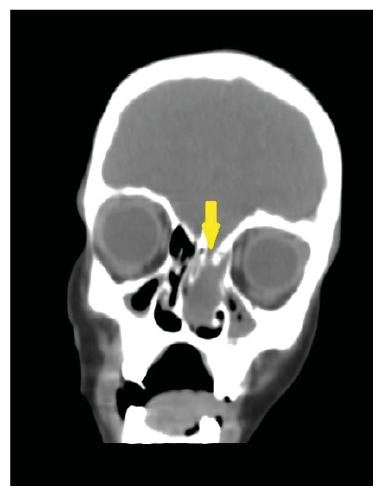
On coronal reformated CT images, a bony defect is seen on left lamina cribrosa. (yellow arrow). Heterogenous image of encephalocele and mucosal tissue is seen on the left ethmoid sinus and nasal cavity.
